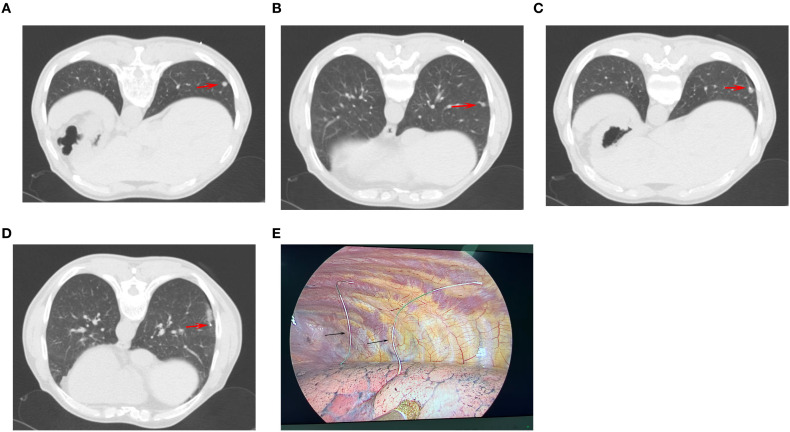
(A, B) The CT-guided SHA localization for PNs (arrows) in the right lower lobe. (C, D) The SHAs (arrows) were placed near the PNs for localization. (E) The SHAs (arrows) were detected during the VATS.
